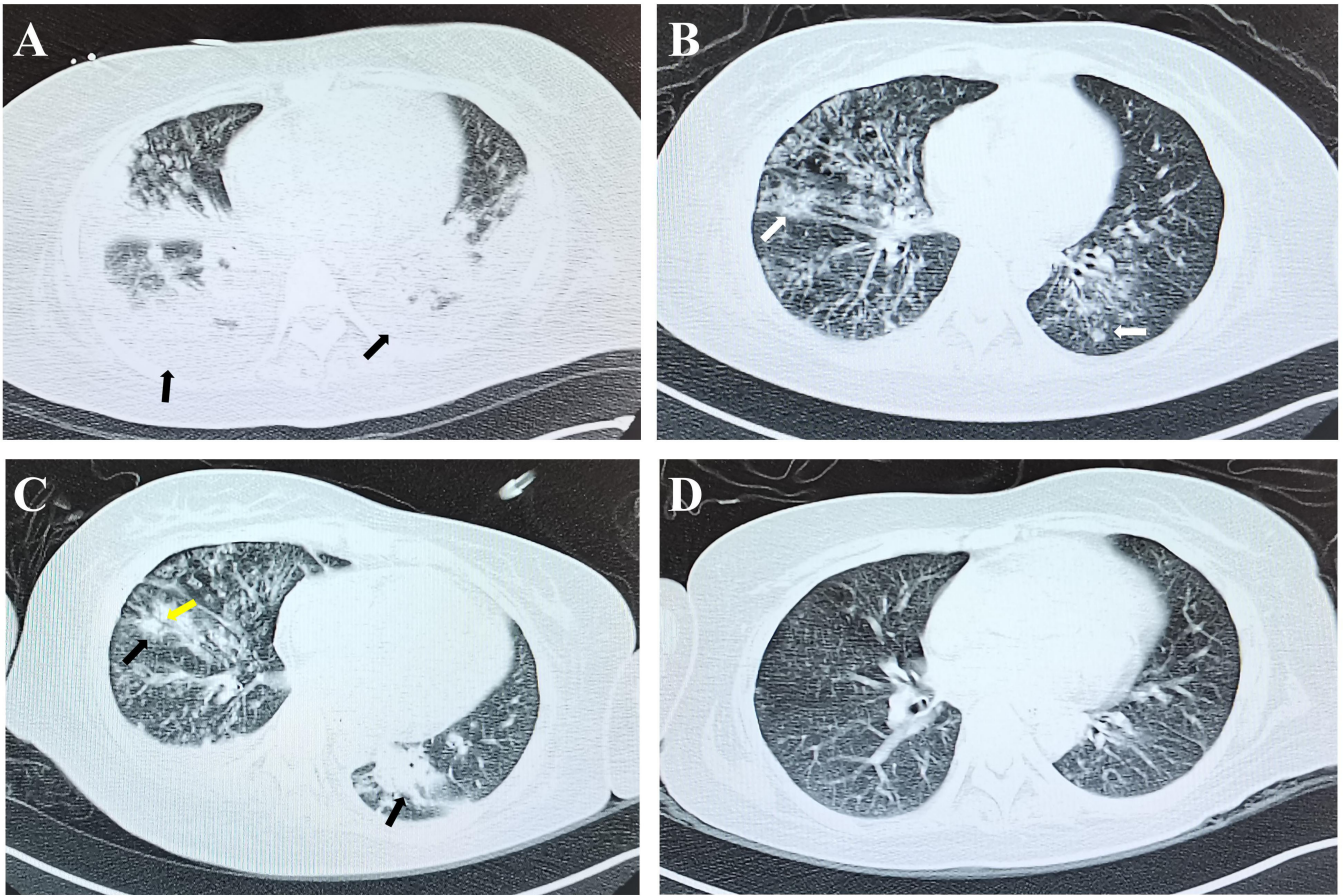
Patient’s chest CT results. Upon admission, the patient’s chest CT revealed lung consolidation (black arrows, (A). On the eighth day of ICU admission, the consolidation resolved, presenting as ground - glass opacities (white arrows, (B). On the 18th day of ICU admission, the patient’s condition had deteriorated again. The consolidation in both lungs had increased (black arrows), and air-space consolidation had emerged (yellow arrow, (C). On the 60th day of ICU admission the patient’s lungs were normal (D).
